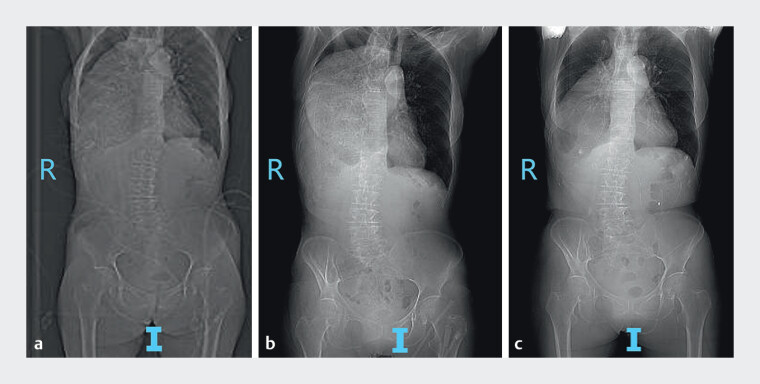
Plain radiographs of the chest and abdomen.
